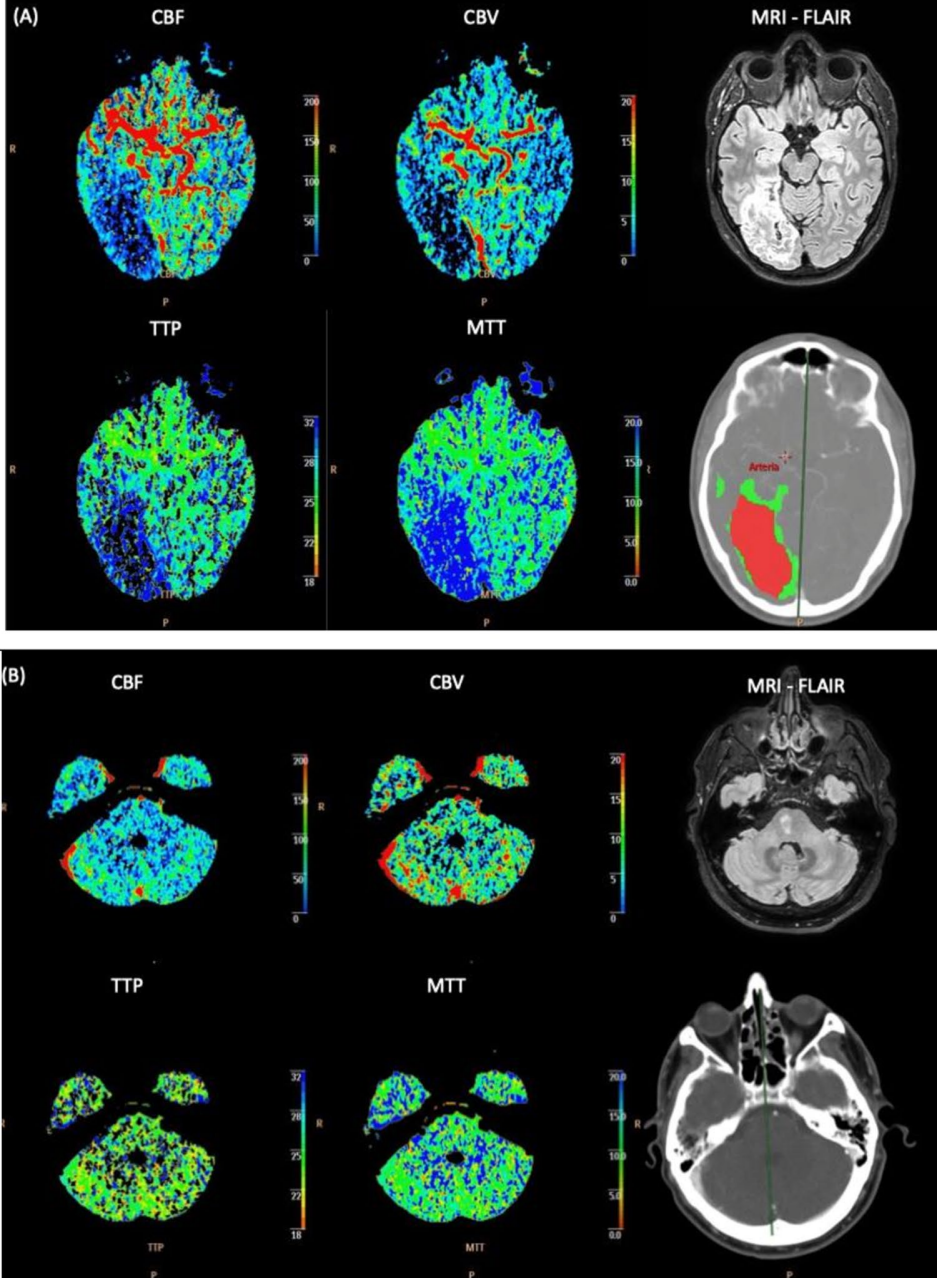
A A 43-year-old man with a history of atrial fibrillation presented to the emergency department about an hour after the onset of headache, visual field deficit, vertigo, unsteady gait, and left hemiparesis (NIHSS 10). At NECT, there was evidence of a blurred loss of cortico-subcortical differentiation in the right occipital area (ASPECTS 9); at CTA, there was occlusion of the P2 tract of the right posterior cerebral artery. Finally, evidence of increased rates in MTT and TTP and decreased rates in CBF and CBV was found at CTP in the right occipital area compatible with a large core and minimal penumbra. Control MRI performed 5 days after the acute event confirmed the known ischemic lesion. B A 42-year-old man, with a history of dyslipidemia, presented to the emergency department about 3 h after the onset of gait ataxia, hypoesthesia in the face, and left upper limb and mild dysarthria (NIHSS 2). At NECT, CTA, and CTP, there was no evidence of alterations in the acute phase. Control MRI performed 5 days after the acute event documented a 15 mm × 8 mm ischemic vascular lesion in the right paramedian pons
